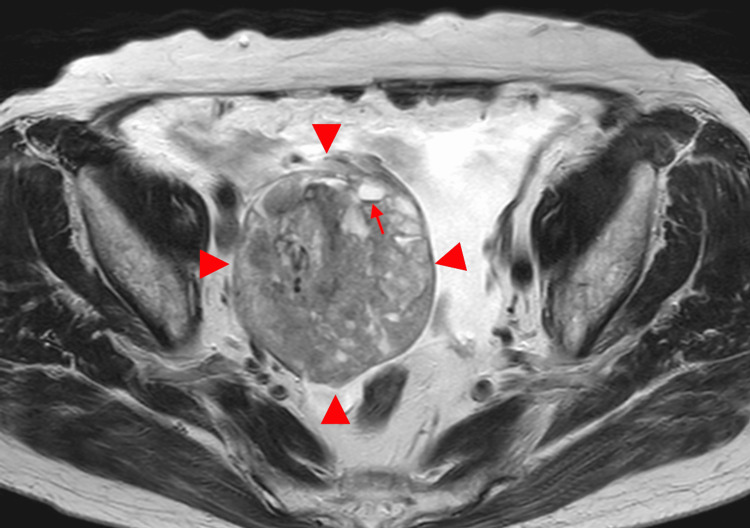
This is the same patient as shown in Figure 4. The axial T2-weighted image demonstrates a well-defined solid mass with heterogeneous signal intensity and partial cystic components (arrowhead). Areas of low signal intensity and fluid levels are noted within the mass (arrow).
